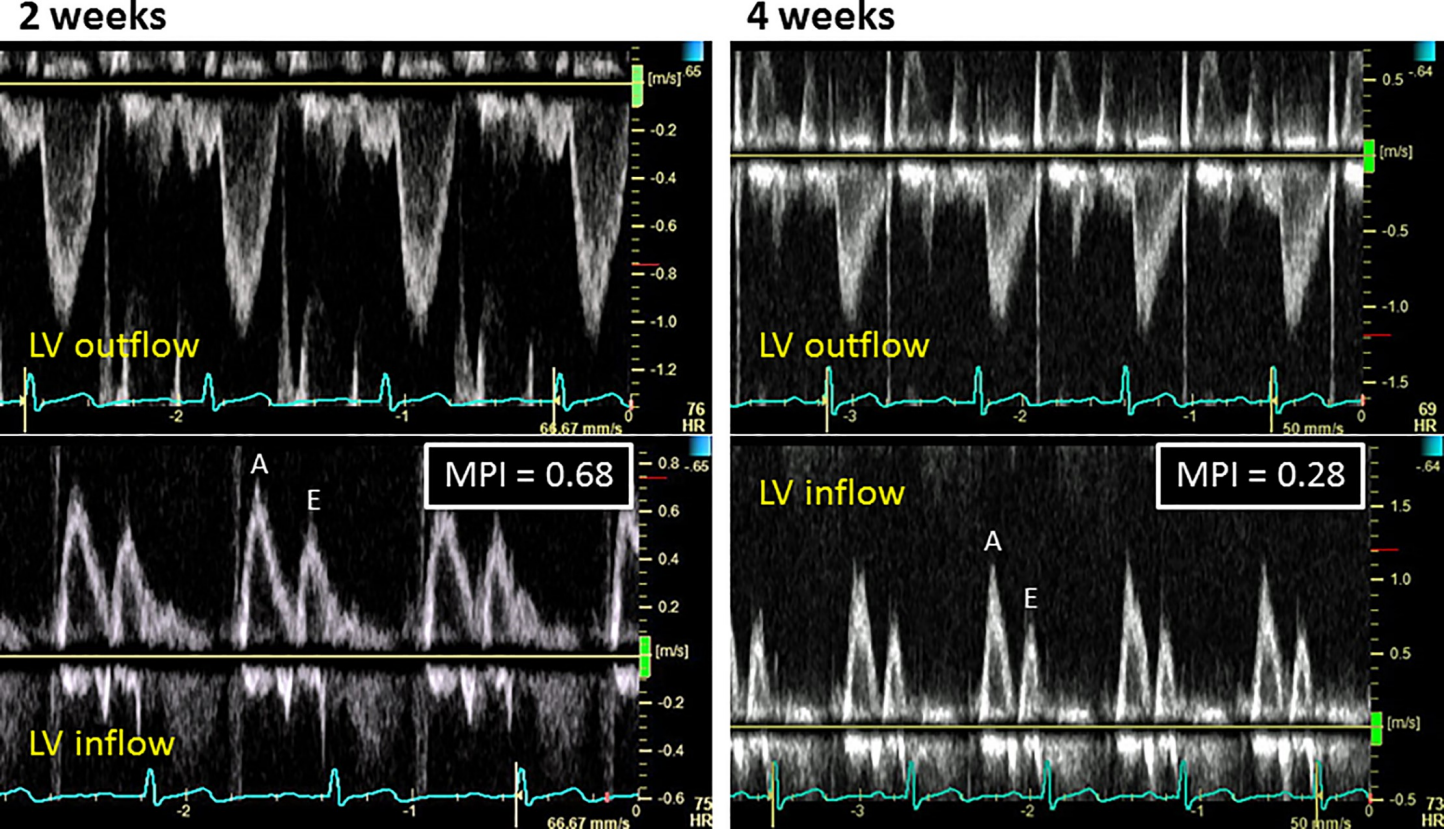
Doppler LV outflow (top) and inflow (bottom) profiles used to measure MPI at 2 and 4 weeks after diagnosis of IVI in a woman aged 42 years.
